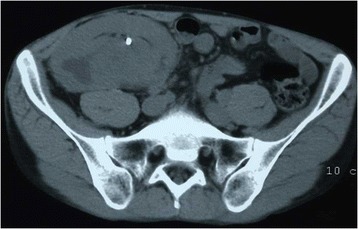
Abdominal computed tomography (CT) scan of the patient. Abdominal CT scan prior to surgery showed a mass measuring 6.4 × 3.7 cm in the transplanted kidney. A double-J ureteral stent was placed.
